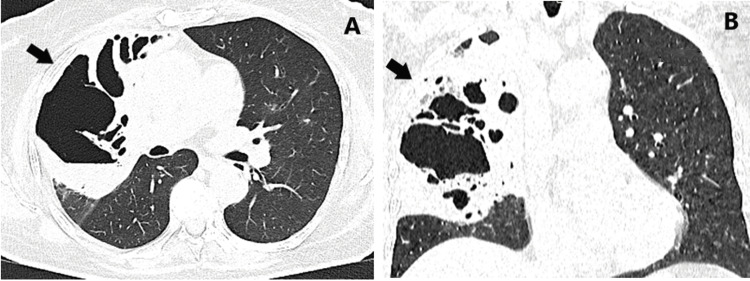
Chest CT in axial (A) and coronary (B) axis, presenting an abscess cavity (arrows) with destruction of the nearby parenchyma.
